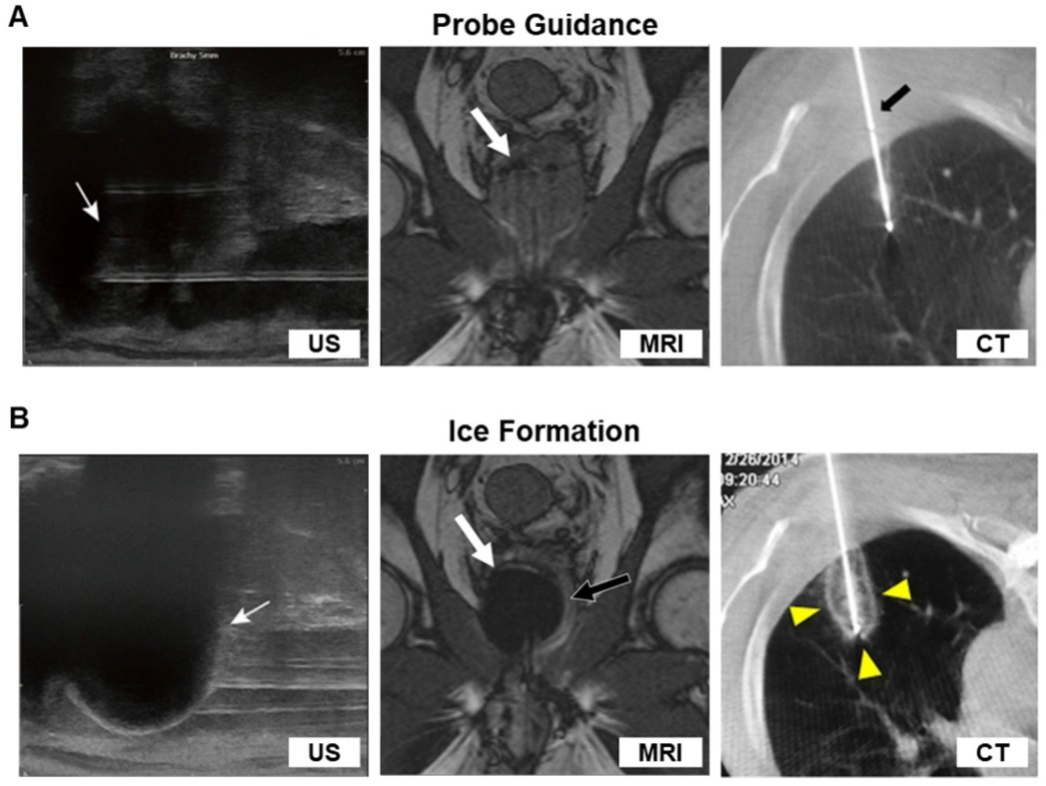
Pre-ablation guidance of the cryoprobes and real-time images of ice formation. (A) Ultrasound (US) image shows hyperechoic delineation of the probe and hypoechoic tumor (noted by arrows). In both the MR and CT image, the cryoprobe was brightly enhanced against the background. The arrows in the MR and CT images indicated the probes. (B) US image showed strong acoustic posterior shadowing by the ice ball. Likewise, T1-weighted MR image outlined the hypointense ice ball, and the CT scan showed ice ball formation (arrowhead) at the end of the cryoprobe. The US, MR, and CT images were adapted and reprinted under terms of the CC-BY license from ref. 162, 163, and 164, respectively. Copyright, 2017. Springer Nature. Copyright, 2019. The British Institute of Radiology. Copyright 2016, Springer Nature.
